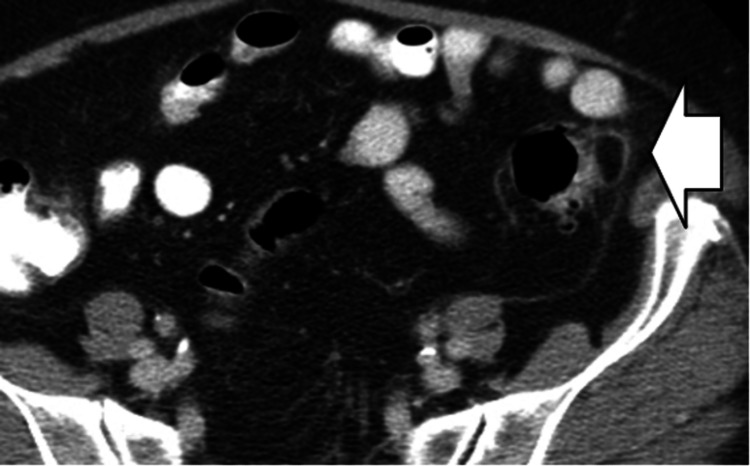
Epiploic appendagitis. CT image shows soft tissue stranding around the epiploic appendage (white arrow) extending off the sigmoid colon.
